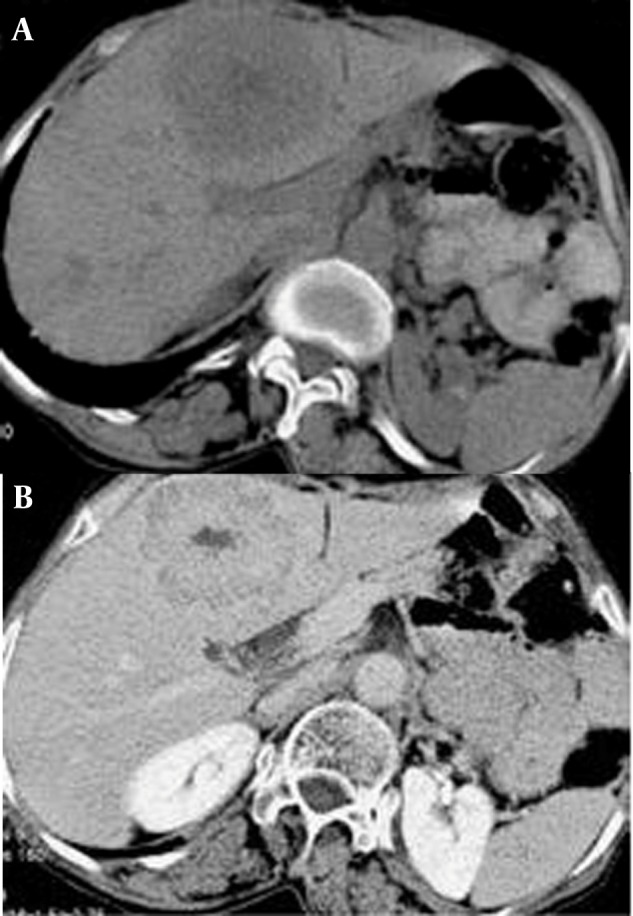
Helical CT in hepatocellular carcinoma A, Hepatocellular carcinoma. Nonenhancing helical CT shows a low density structure (lesion) with an ill-defined border in the left hepatic lobe; B, Hepatic parenchymal phase of helical CT shows a solid enhanced lesion with central scar.
